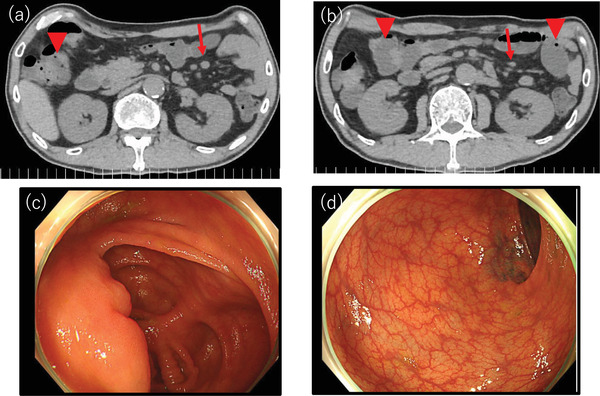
Computed tomography scan of the abdomen: an accumulation of fluids in the small bowel (arrowhead) is noticeable. (a,b) Intra‐abdominal lymphadenopathy with a maximum diameter of approximately 10 mm is observed (arrow). (c,d) Colonoscopy: no obvious anomalies are noted
